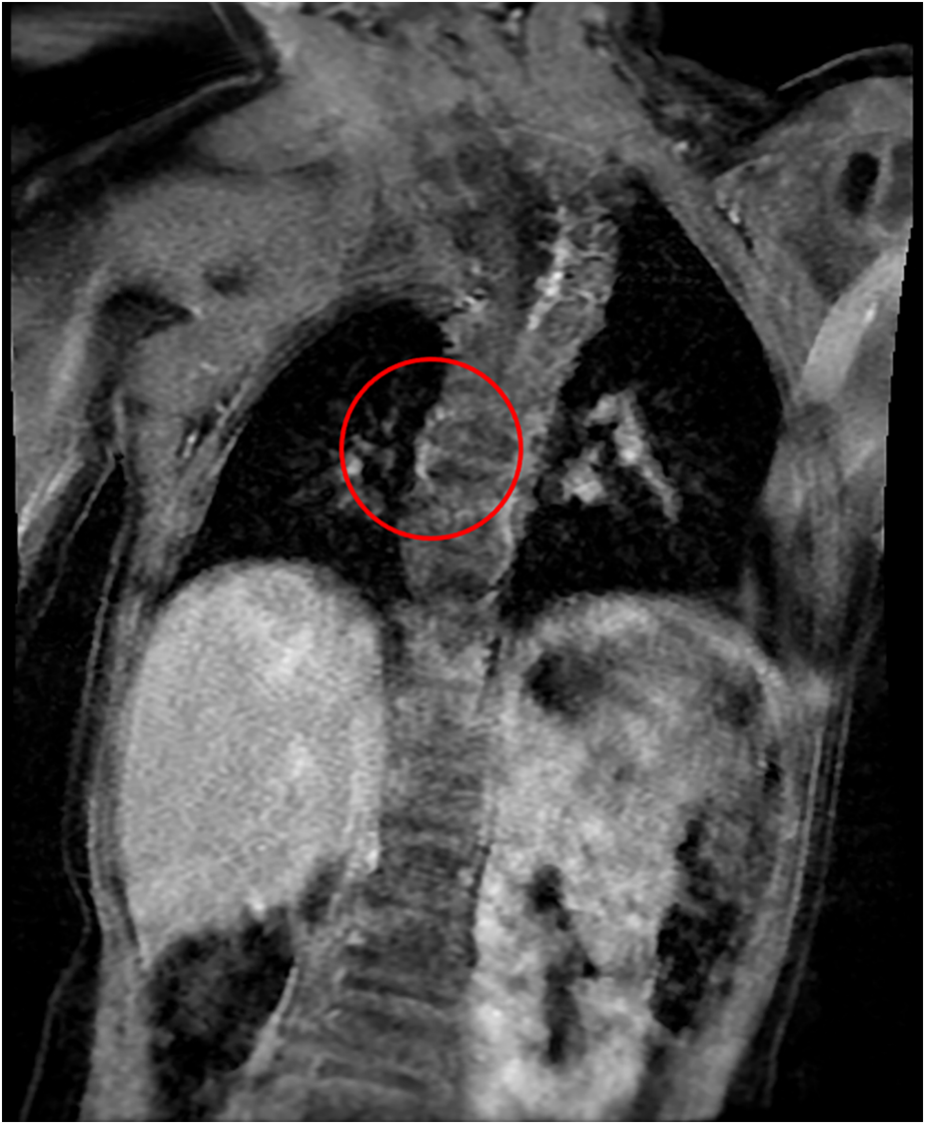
MRI scan 16 months after surgery (the circle shows no lesions).
